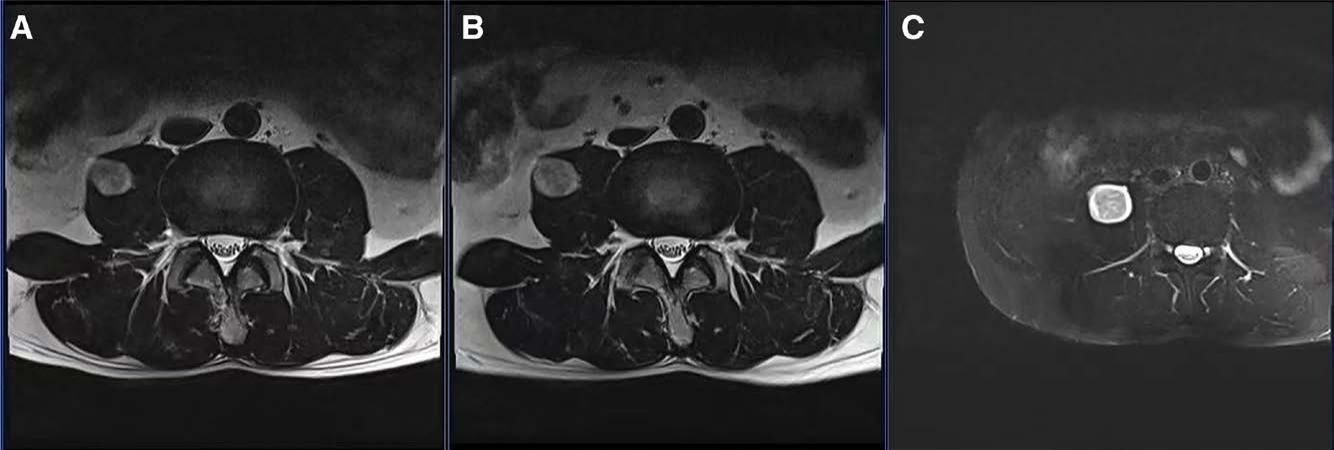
The MRI images. Panel (A) shows a slightly longer T2 signal with a diameter of about 1.7 cm in the right psoas muscle; panel (B) shows a slightly longer T2 signal with a maximum diameter of about 1.9 cm in the right psoas muscle; panel (C) shows a signal of about 30 mm × 32 mm × 40 mm T1/T2 slightly longer with clear border.
